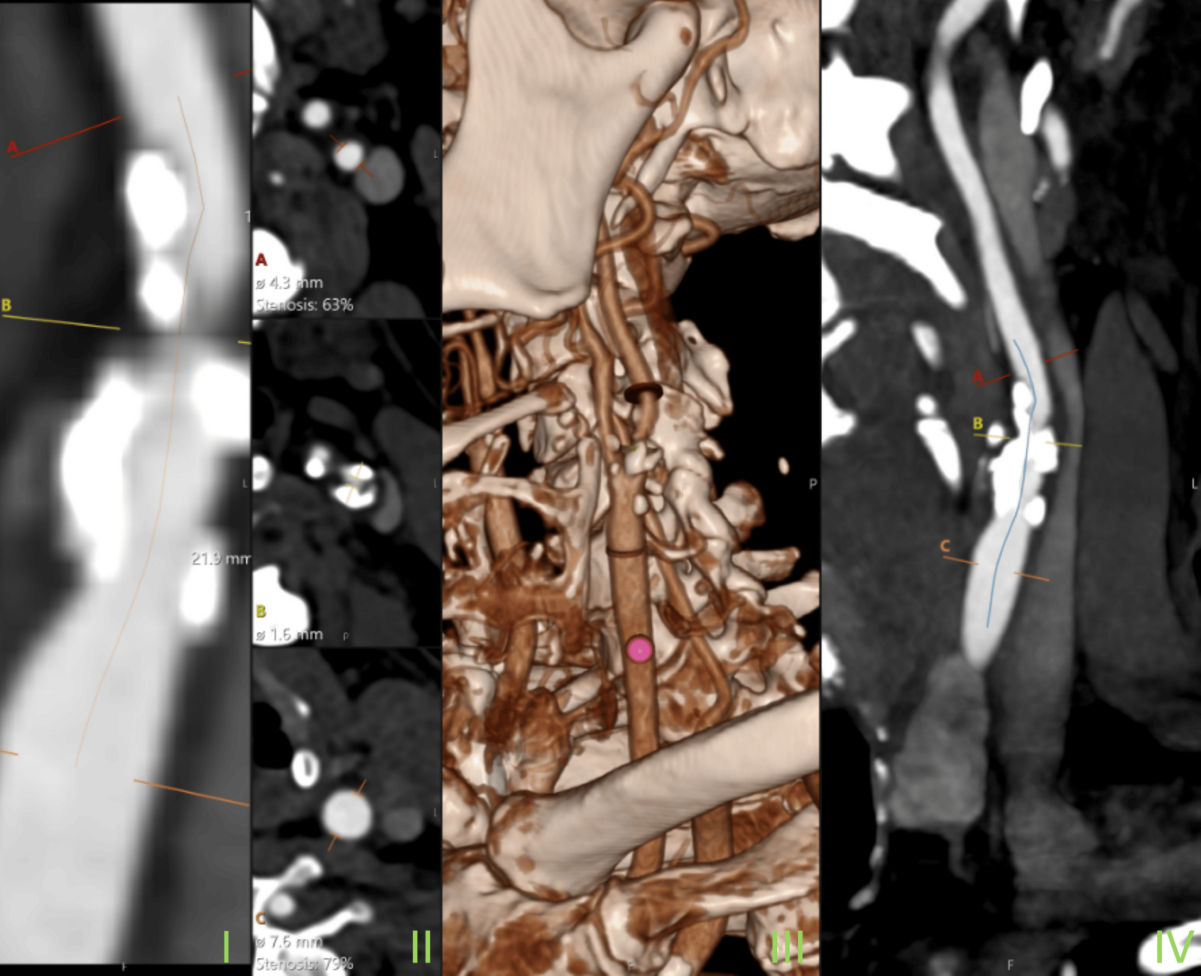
CT angiogram of the left carotid artery. The slide labelled I shows a longitudinal image of the left carotid artery, demonstrating atherosclerotic plaque buildup and luminal narrowing. Marked line A (red) shows the distal measurement point. Line B (yellow) shows the point of maximal stenosis, and line C (orange) shows the proximal reference point. The orange line shows the length of the stenotic segment which is 21.9 mm (orange Line). The slide labelled II shows cross-sectional views of the artery at three levels (A, B, and C), matching locations in Slide I. At level A (red), the luminal diameter is 4.3 mm, corresponding to 63% stenosis. At level B (yellow), the luminal diameter is 1.6 mm, representing the most stenotic region, and at level C (orange), the luminal diameter is 7.6 mm, resulting in 79% stenosis. Slide III shows a 3D reconstruction of the carotid and vertebral arteries and surrounding anatomies and highlights the same stenotic levels, i.e., A (red), B (yellow), and C (orange). Slide IV shows a longitudinal view tracing the entire course of the left carotid artery. Levels A, B, and C are labelled to correlate with images as described in previous slides. The blue curved line represents the center line of the artery for planning reconstruction.
